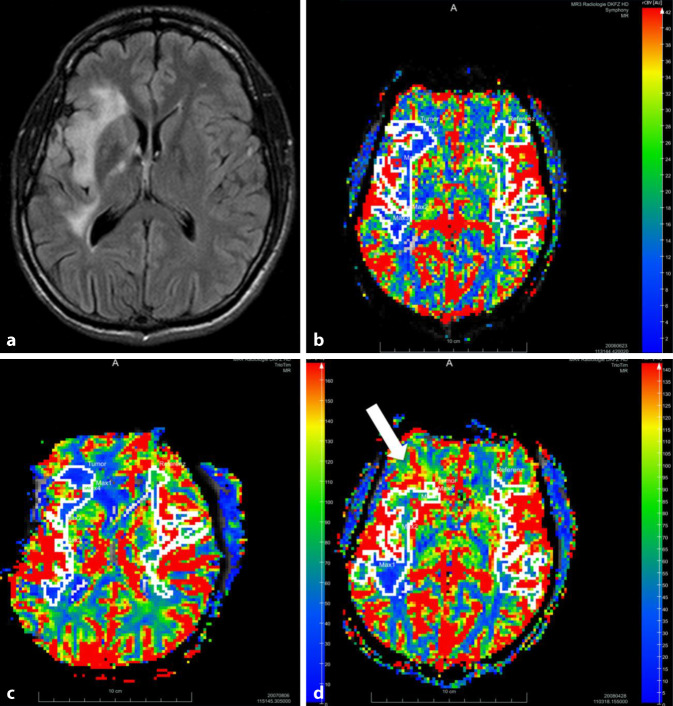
Surveillance with perfusion MRI. A 37-year-old patient with WHO grade II astrocytoma in the right hemisphere visualized on representative axial FLAIR (a) image at baseline. DSC-rrCBV maps at baseline (b) and at the 14-month follow-up (c) do not show elevated rrCBV values in the tumor region. At the 20-month follow-up (d), the time point of progressive disease as determined by RANO criteria, rrCBV elevation is seen in the right frontal and insular subcortical white matter (white arrow)
